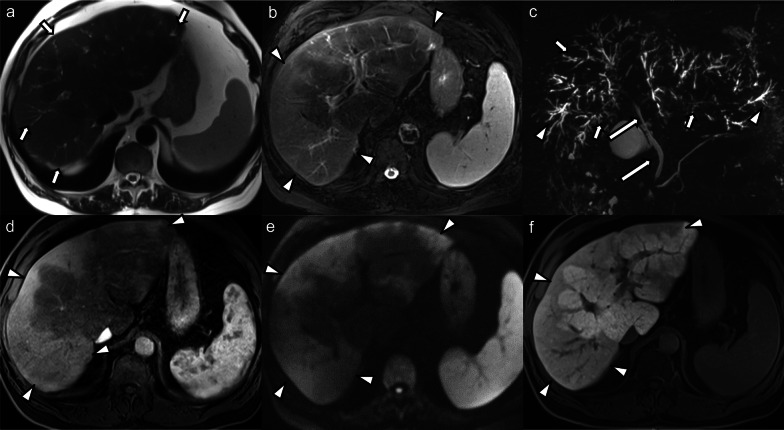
70-year-old male patient who developed generalized pruritus and laboratory signs of hepatopathy a few months after recovering from a severe COVID-19 infection. MRI and MRCP of the liver with gadoxetate disodium was performed: a Axial T2-weighted image shows multifocal peripheral areas of mild biliary ductal dilatation and faint hyperintense parenchymal signal changes (arrows). b Peripheral parenchymal hyperintense signal changes are better seen on the fat-suppressed T2-weighted image (arrowheads). c Maximum intensity projection MRCP shows multiple strictures of the intrahepatic bile ducts (arrows) with upstream dilatation (arrowheads). The extrahepatic bile duct is spared (long arrows). d Post-contrast image in the arterial phase shows hyperenhancement in the peripheral parenchymal areas with hyperintense signal on high b-value diffusion-weighted images (e) and hypointensity in the hepatobiliary contrast phase (f) (arrowheads in d–f). Biopsy showed findings consistent with chronic cholestatic hepatopathy, bile duct damage and Stage F3 fibrosis
